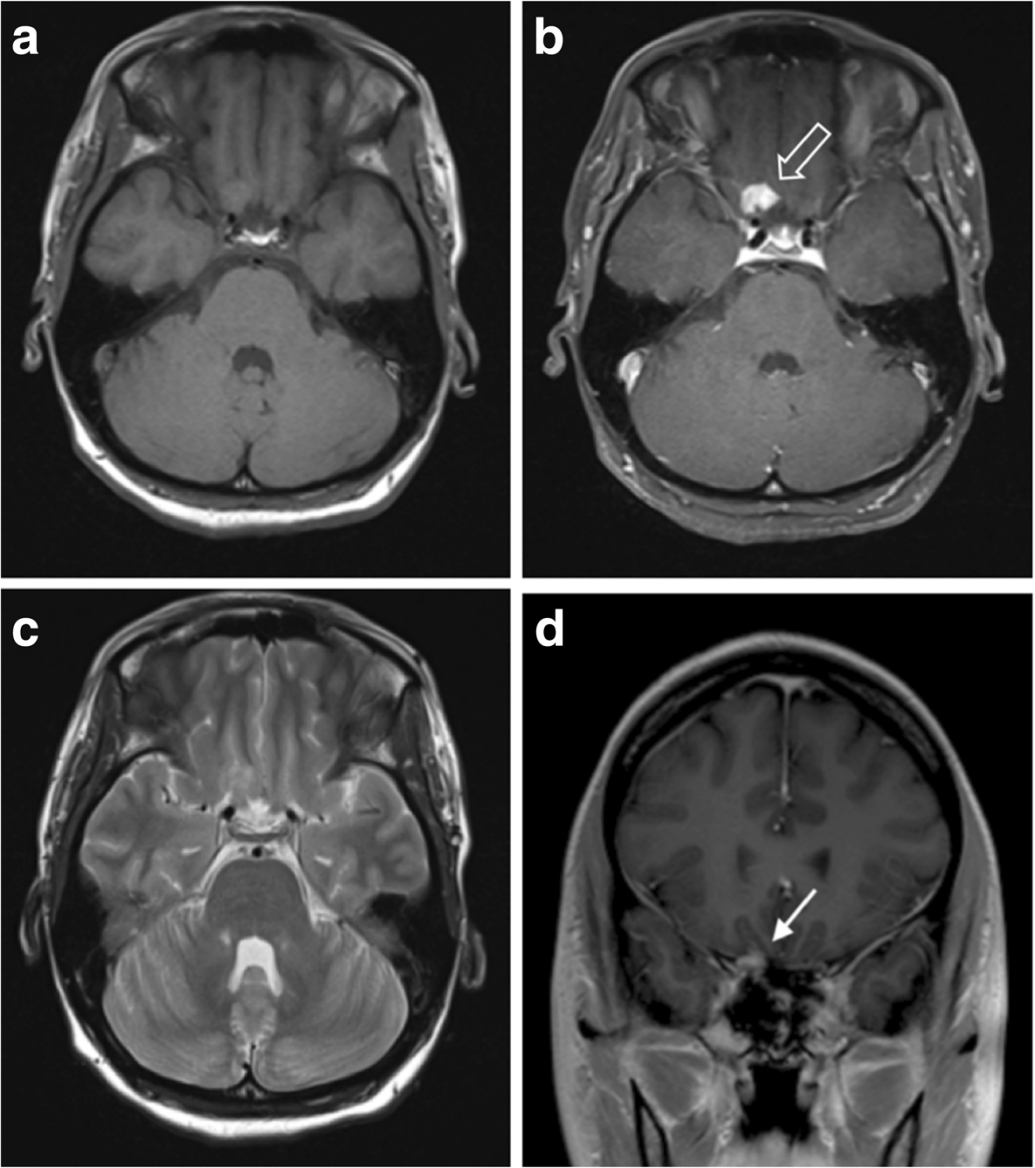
Solitary tuberculoma. a, b Pre- and post-contrast axial T1-weighted MR images showing a strongly and homogenously enhancing lesion with a wide dural base at the right orbital apex (outlined arrow). No other intracerebral or meningeal lesions were seen. c T2-weighed axial MR image of the same lesion showing an isointense lesion with no associated vasogenic oedema. d Post-contrast coronal T1-weighted MR image demonstrating the lesion’s dural attachment (solid white arrow)
